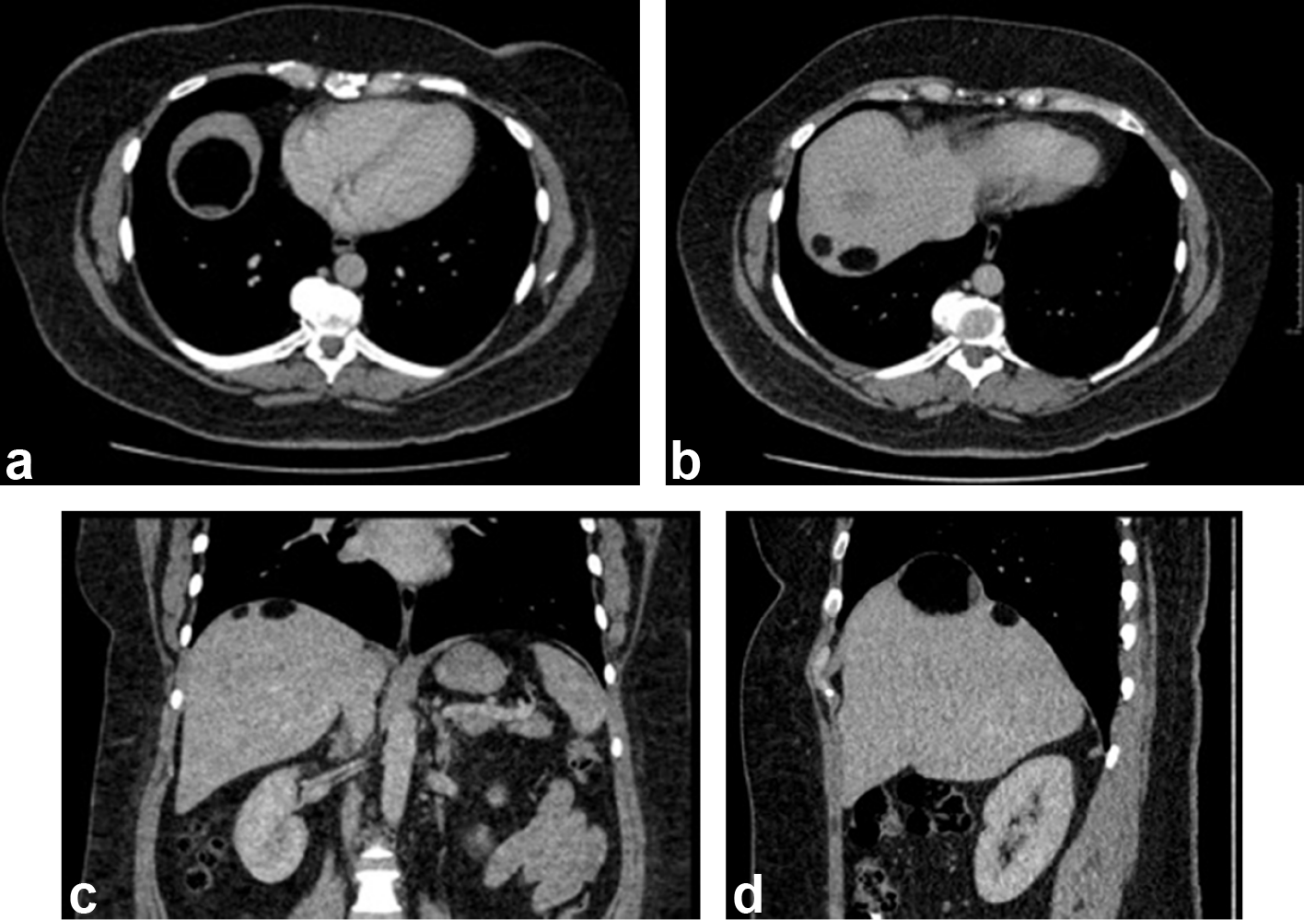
(a, b) Axial, (c) coronal and (d) sagittal: these images from CT abdomen in portal venous phase shows right upper quadrant lesions containing macroscopic fat, soft tissue and calcification.
